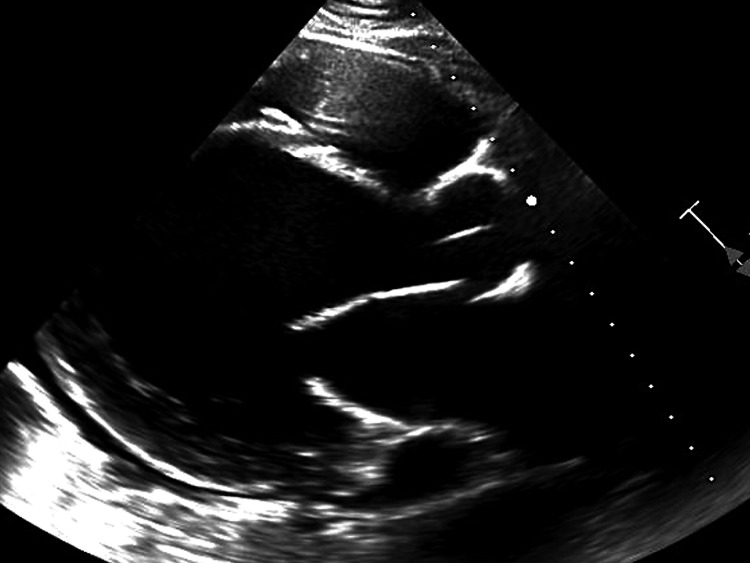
Transthoracic echocardiogram Parasternal long view demonstrating severe dilatation and global hypokinesis of the left ventricular cavity with an ejection fraction of 10% and mild pericardial effusion
